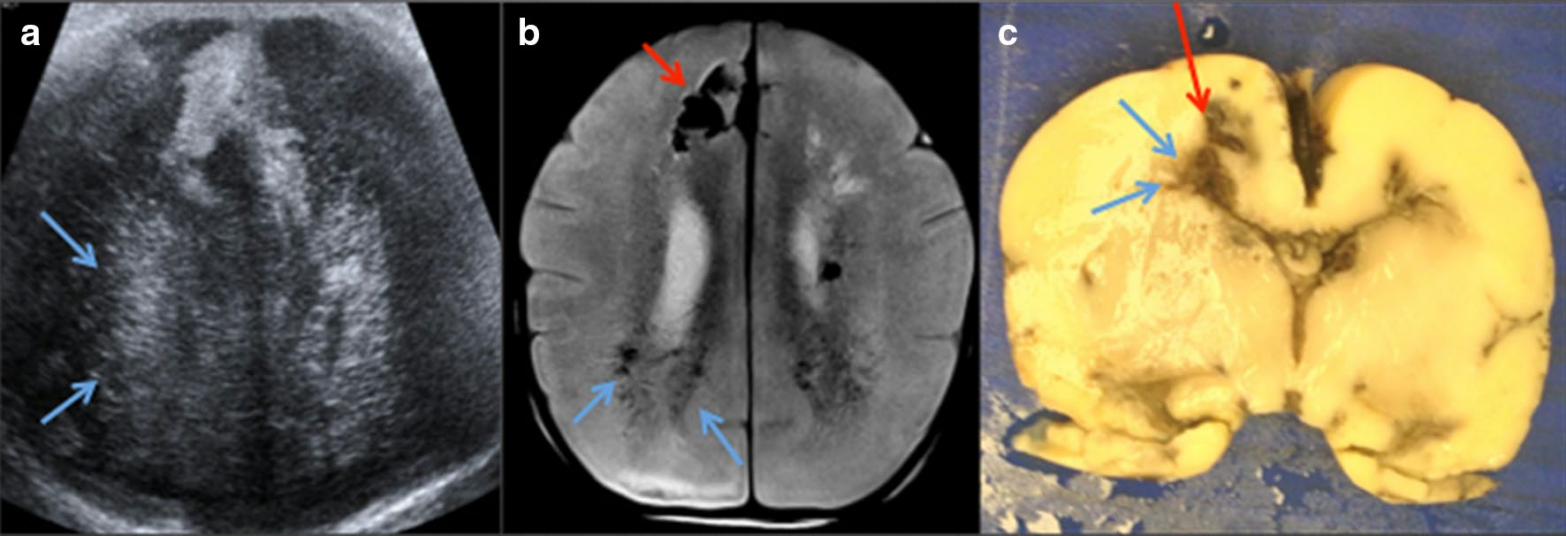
Intracranial haemorrhage in a 29-week premature newborn. a Ultrasound, (b) axial T2W MRI, (c) pathology showing periventricular (blue arrows) and frontal haemorrhage (red arrow)
